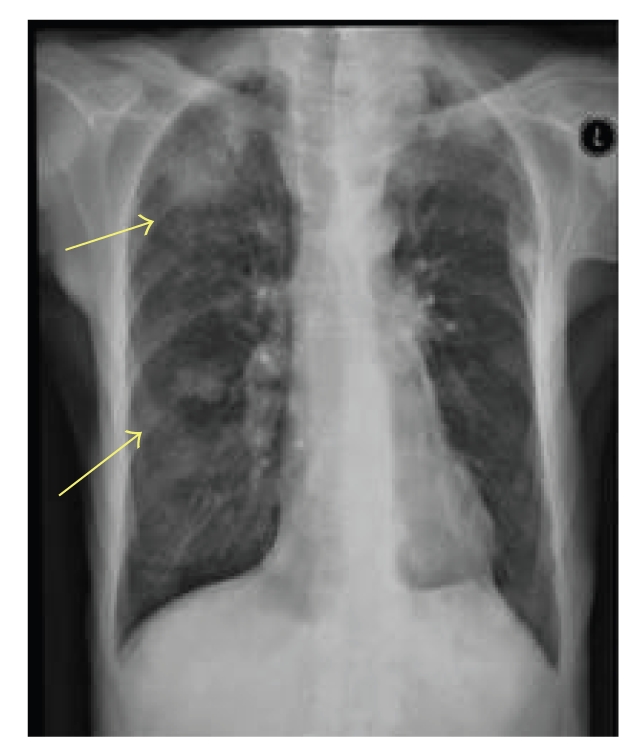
Chest radiograph showing multiple lung metastases.
